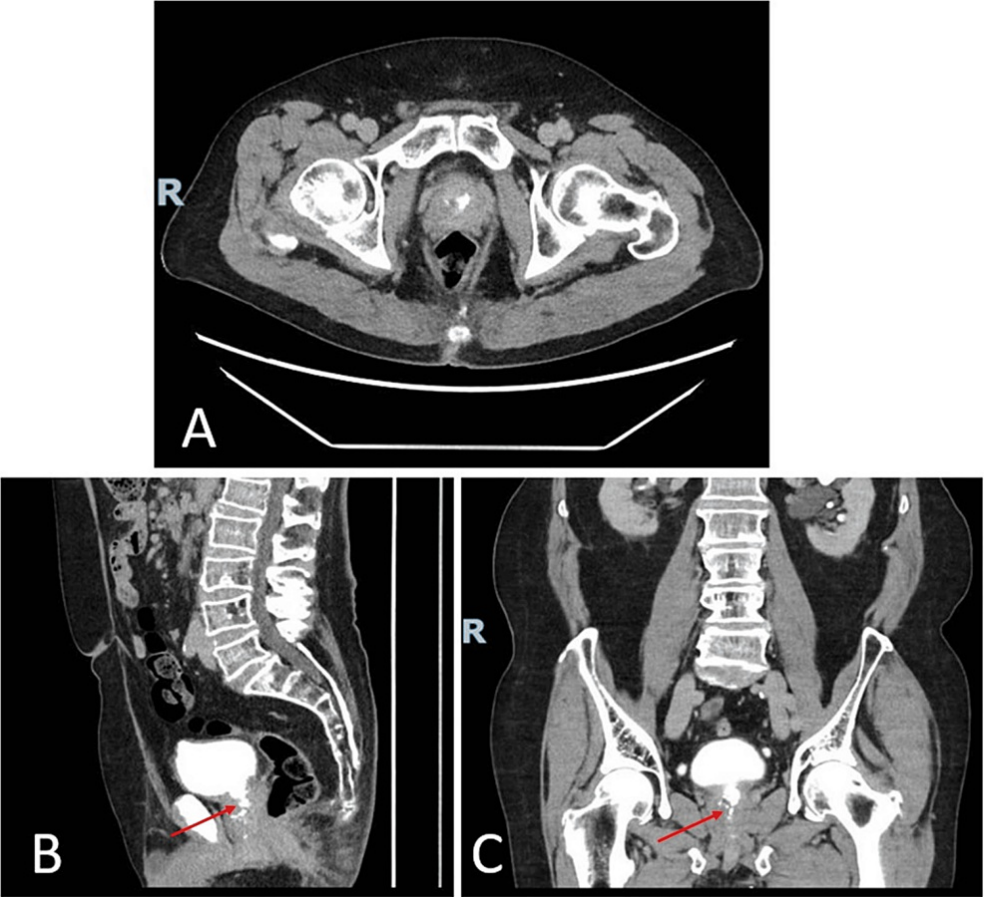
Axial (A), sagittal (B), and coronal (C) views of the CT of the chest, abdomen, and pelvis with urogram demonstrating a TURP defect of the prostate (red arrows) prior to starting combined chemoradiation.
